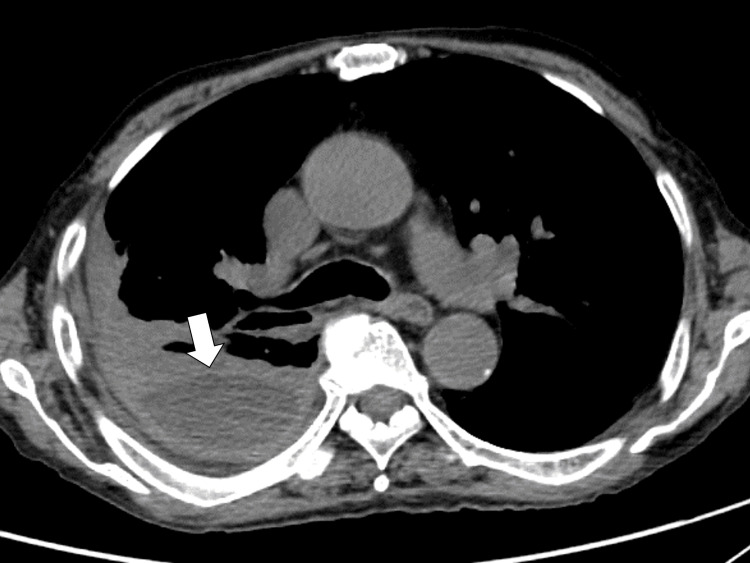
Chest CT showing right capsulated chest effusion (white arrow). CT: computed tomography.
